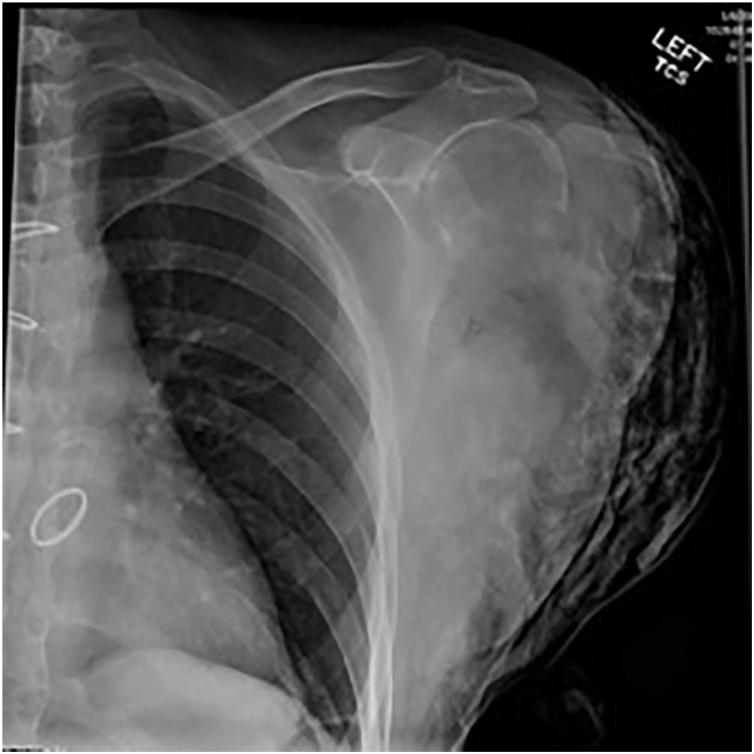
Post-operative anterior-posterior radiograph of the left shoulder.
